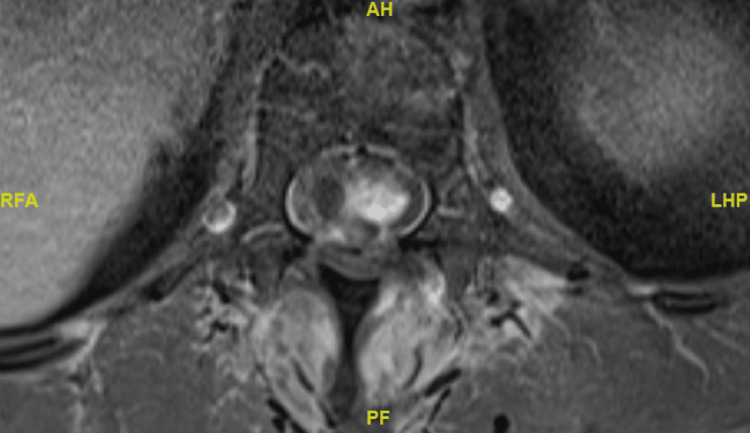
Post-operative axial MRI image of intradural lesion at the level of T12 with a notable absence of metal artefacts.
